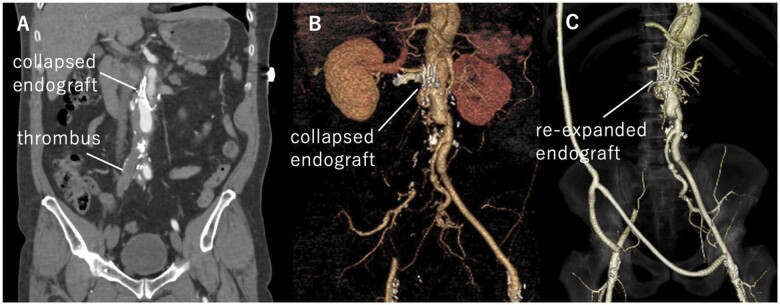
Endograft re-expansion before and after extra-anatomic bypass. Preoperative computed tomography (A) coronal image, (B) 3D image and (C) postoperative 3D computed tomography.
